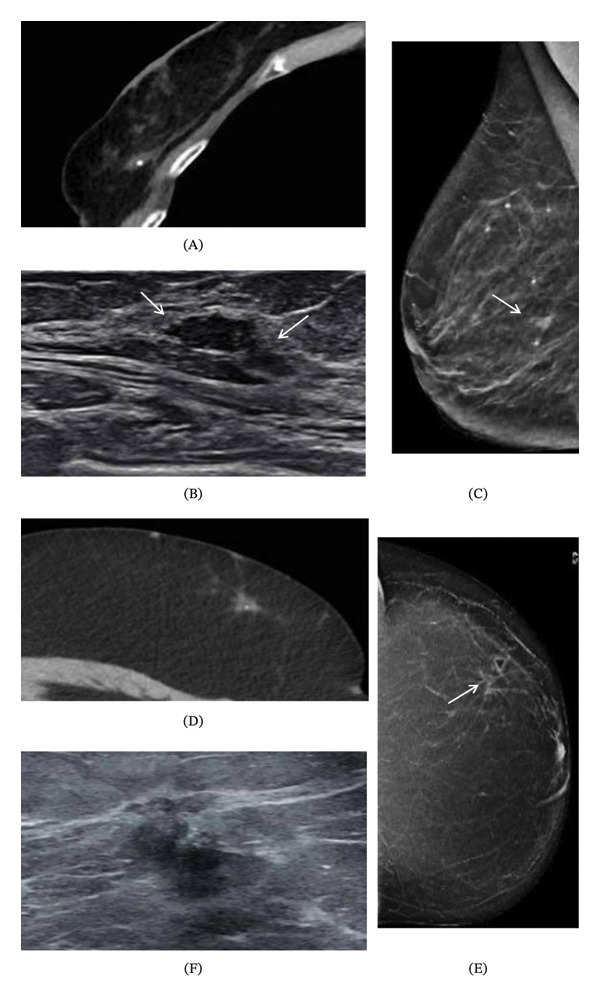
Known malignancies incidentally visualized on chest CT. (A) Chest CT demonstrating small soft tissue density oval mass (white arrow) in the right lower outer quadrant with biopsy clip in place, with biopsy results reported as consistent with invasive ductal carcinoma. (B) Prebiopsy ultrasound demonstrating a hypoechoic irregular mass without circumscribed margins which was felt to correspond with the CT finding. (C) Prebiopsy mediolateral oblique (MLO) view mammogram of the right breast demonstrating irregular mass at the 9 o’clock position (white arrow). (D) Chest CT demonstrating soft tissue density spiculated mass with associated punctate calcifications in the left breast upper outer quadrant (white arrow). (E) Grayscale ultrasound demonstrating horizontal irregular mass with spiculated margins and associated microcalcifications (white arrowhead) and internal vascularity on color Doppler (not shown). (F) MLO view mammogram of the left breast demonstrating irregular mass with spiculated margins and associated calcifications at the 1 o’clock position (white arrow) which corresponds to the patient palpable area at the site of the skin marker (curved arrow).
